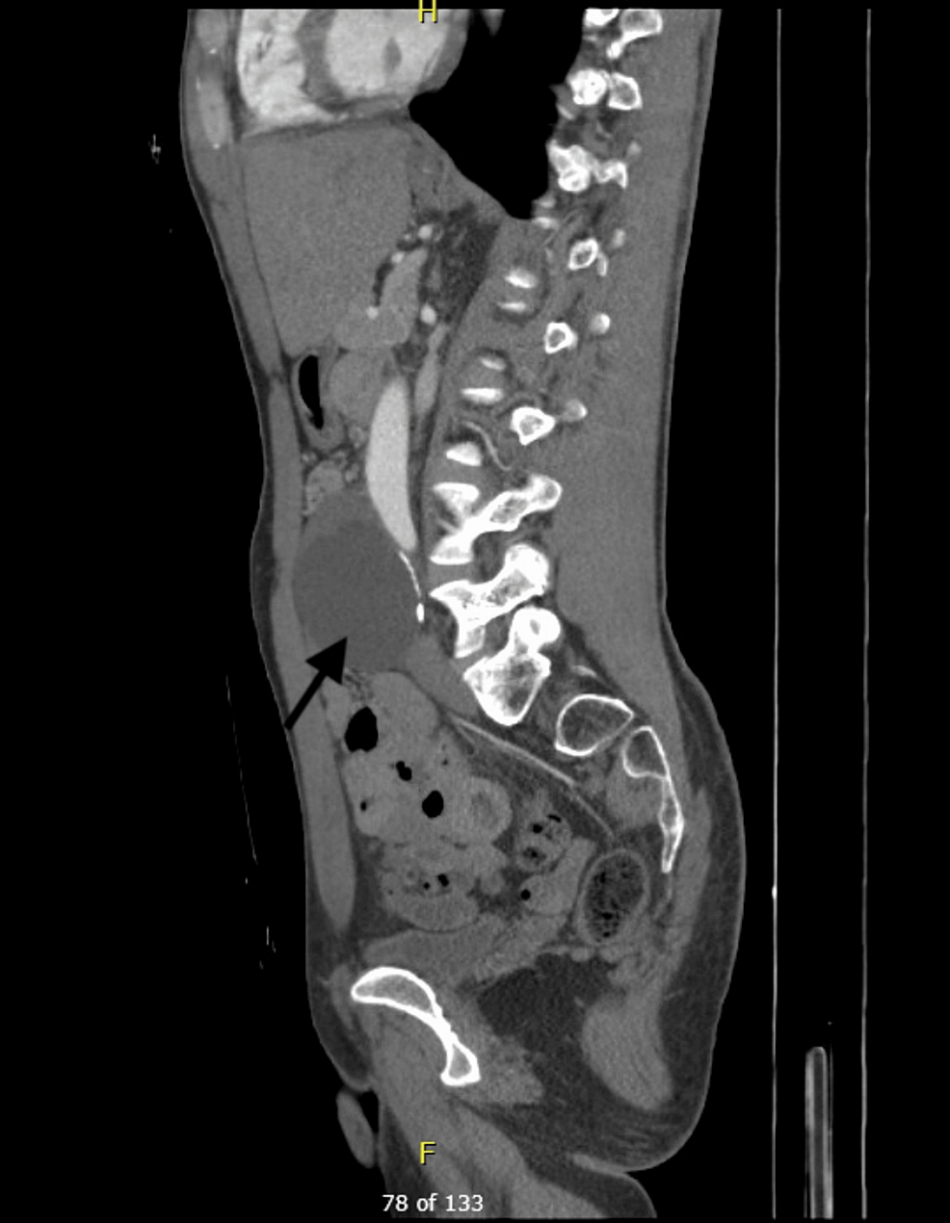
CT scan: sagittal view of para-aortic mass with posterior calcifications
